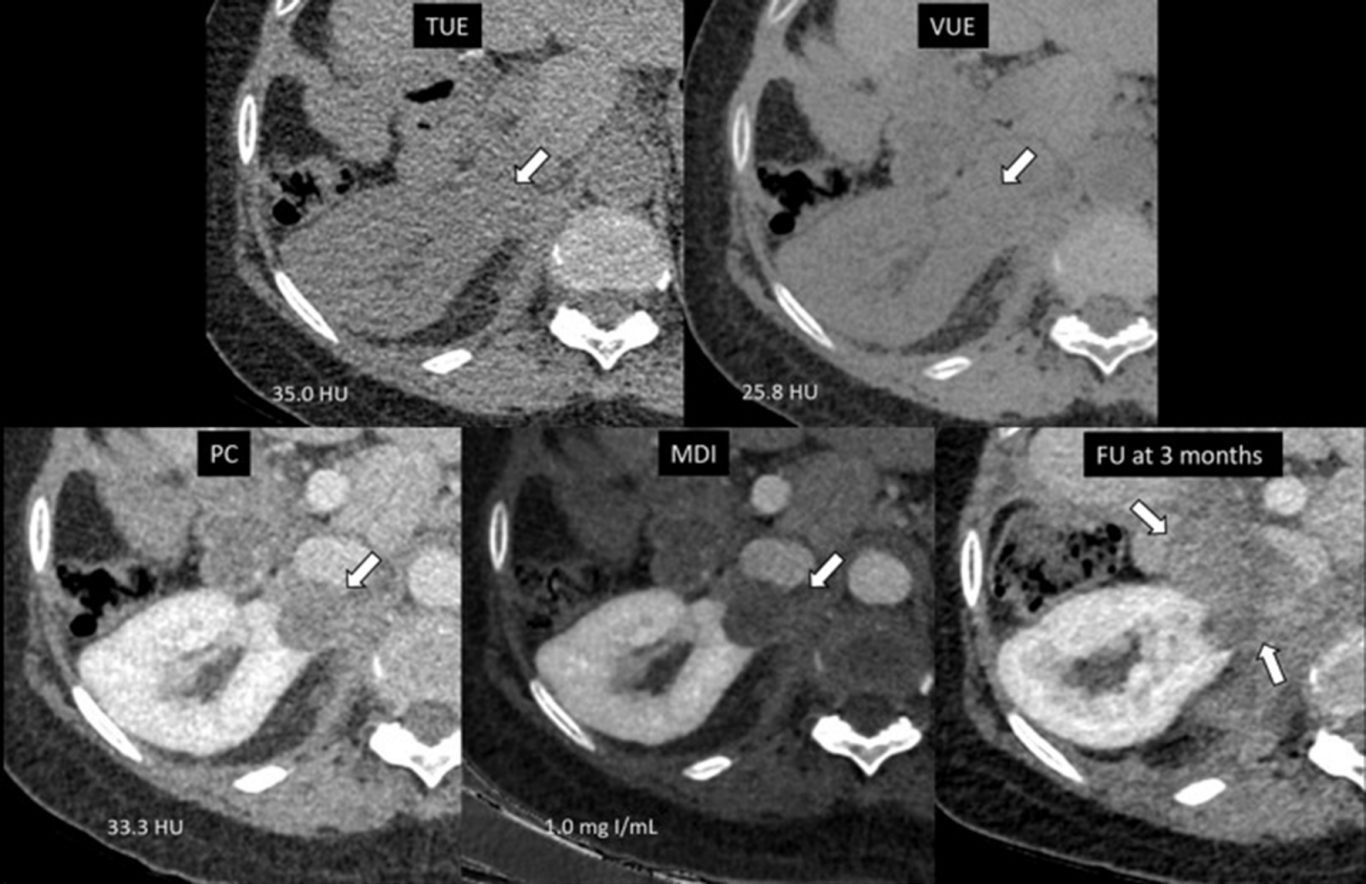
A 2.5 cm enhancing renal mass was incorrectly classified as a cyst due to attenuation differences of less than 20 HU between post-contrast and true unenhanced images, as well as an iodine concentration below 1.6 mg I/mL. This misclassification was likely due to internal necrosis within the mass. However, both readers were able to correctly identify it as a mass with high confidence upon visual assessment. The mass was pathologically confirmed as renal cell carcinoma and has shown size changes on follow-up CT scans. TUE = True unenhanced image, VUE = Virtual unenhanced image, PC = Post contrast images, MDI = Material density iodine images, FU = follow-up
